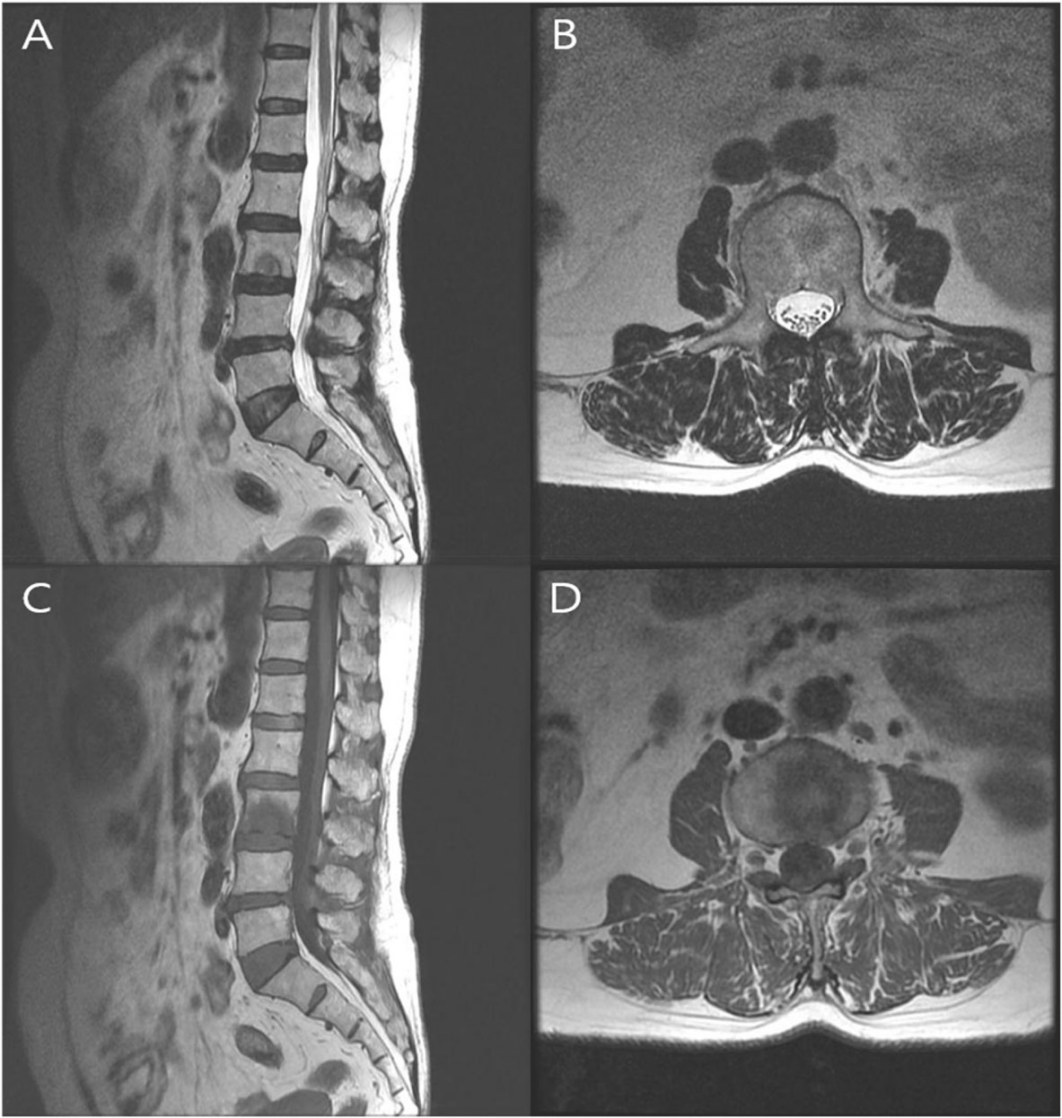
Initial MRI (a) T2 weighted sagittal image and (b) T2 weighted axial view showings Schmorl’s node with iso to high signal intensity, (c) T1 weighted sagittal image and (d) T1 weighted axial image showing low signal intensity
